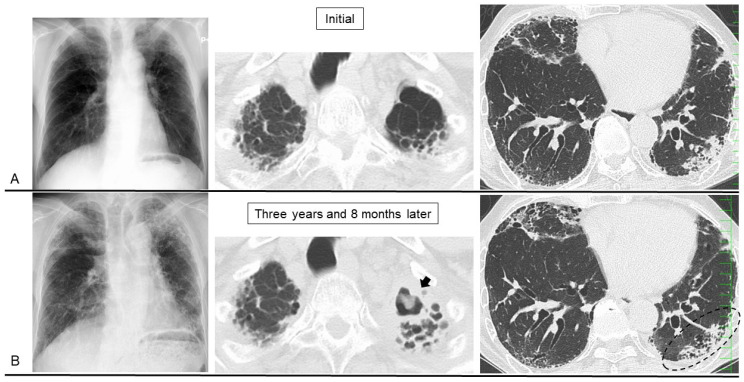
A patient with pleuroparenchymal fibroelastosis in whom the complication of pulmonary aspergillosis developed. ((A): upper row) The initial chest X-ray and computed tomography (CT) images showed pleural thickening, elevation of hilar opacities, and bullae in the upper lung fields. Consolidation with traction bronchiectasis was apparent in the lower lobe. ((B): lower row) Three years and 8 months later (following corticosteroid administration for 3 months), reticular and nodular opacities extended in the bilateral upper lung fields, and hilar opacities were further elevated. CT of the upper lung showed a fungus ball (thick arrow) at the location of the bullae, which was diagnosed as pulmonary aspergillosis. Despite this, the patient’s respiratory symptoms and consolidation shadows on CT partially improved (dashed ellipse) thanks to the corticosteroid.
